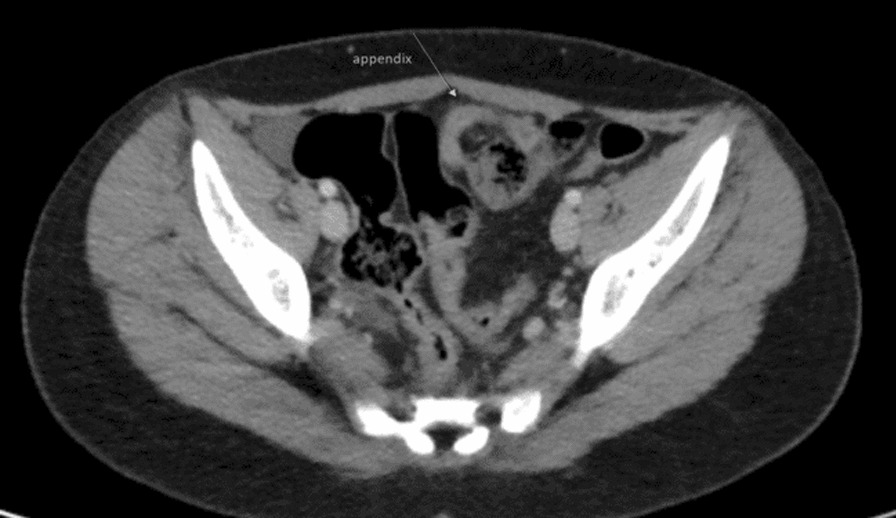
CT of the abdomen (axial cut) showing location of appendix toward the left side of the abdomen
